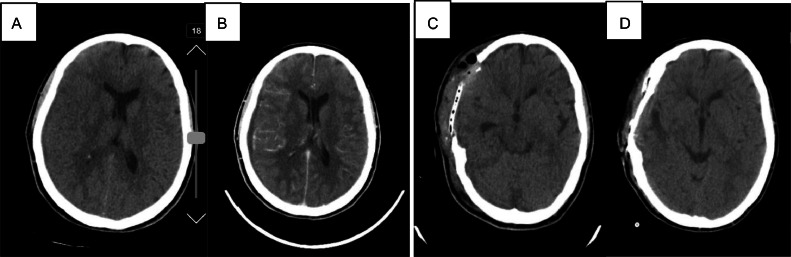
Noncontrast Brain CT scan (A) preoperatively showed a hypodense rim in right frontotemporoparietal with subtle midline shift to the left. Contrast enhanced brain CT scan (B) preoperatively showed a hypodense rim in right frontotemporoparietal with enhancement of adjacent pia matter in favor of a subdural inflammatory collection. (C) Early postoperative CT scan showed right frontotemporoparietal craniectomy, no midline shift with the drainage of a subdural extra-axial fluid collection, which was diagnosed as subdural empyema, (D) One-month postoperatively CT scan showed right frontotemporoparietal cranioplasty with normal brain tissue.
